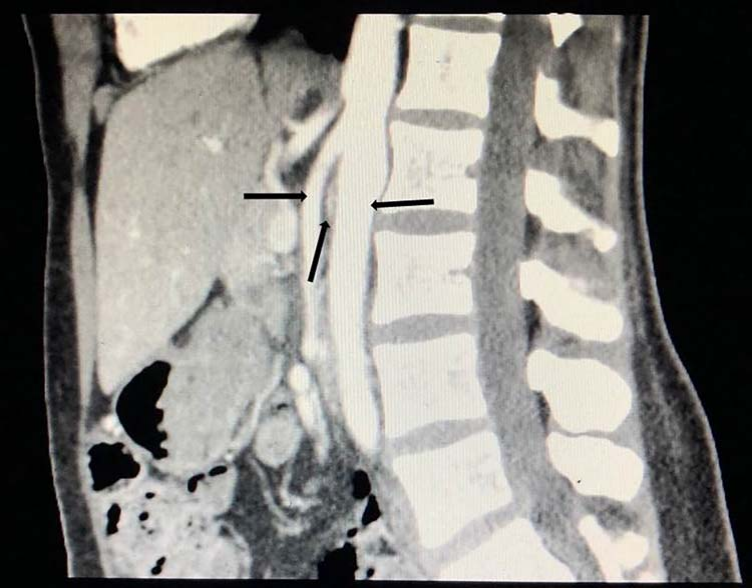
Computed tomography angiogram on presentation, sagittal view showing origin of SMA (left arrow) and the aorta (right arrow) with the compressed LRV in the narrow angle (middle arrow).
